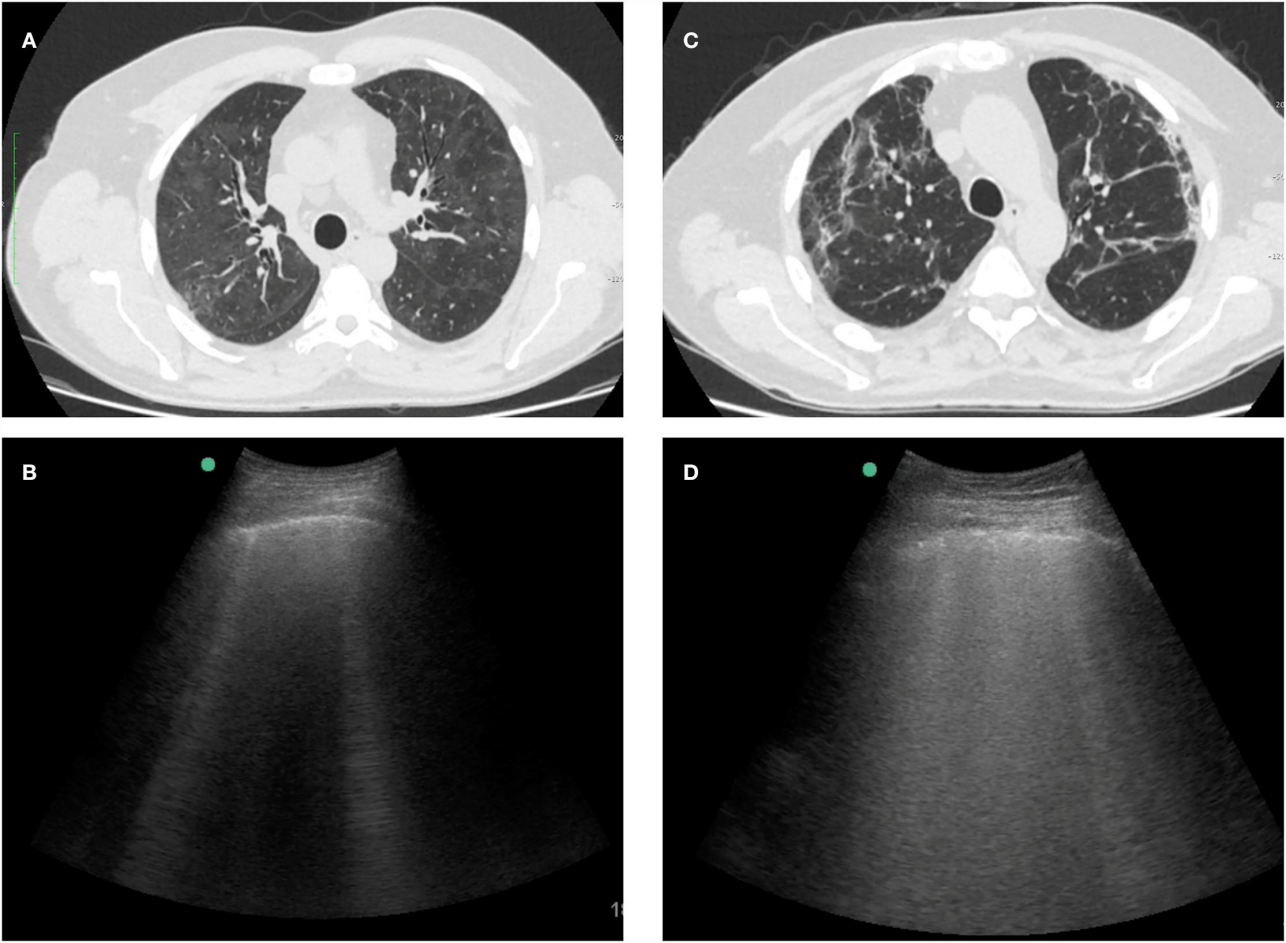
Examples of HRCT and LUS images from 2 patients with interstitial lung sequelae after COVID-19. (A) Persistent diffuse ground-glass opacities on HRCT. (B) LUS of the same patient with isolated B-lines and absence of pleural line abnormalities. (C) Subpleural lines and parenchymal bands on HRCT. (D) LUS of the same patient with confluent B-lines and blurred pleural line.
